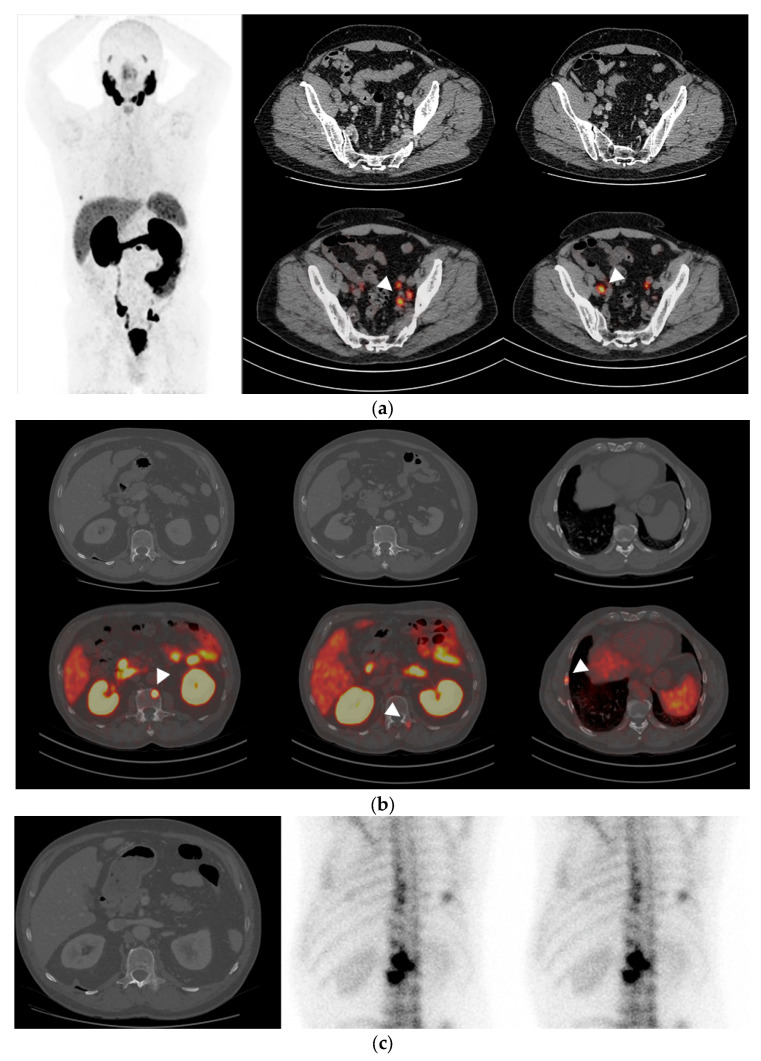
Clinical case: 65-year-old patient, iPSA 28 ng/mL, Gleason score 8 (4 + 4). Multimetastatic nodal (a) and skeletal (b) dissemination (arrowheads) at PSMA-PET/CT, with negative CT (a,b) and bone scintigraphy. (c) PSMA-PET/CT findings were confirmed at subsequent CT and bone scintigraphy exams performed during follow-up.
